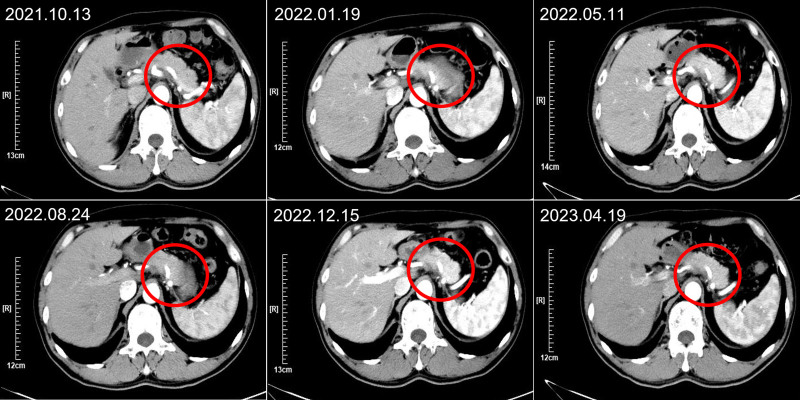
The representative CT images of case 1 after treatment. CT = computed tomography.
